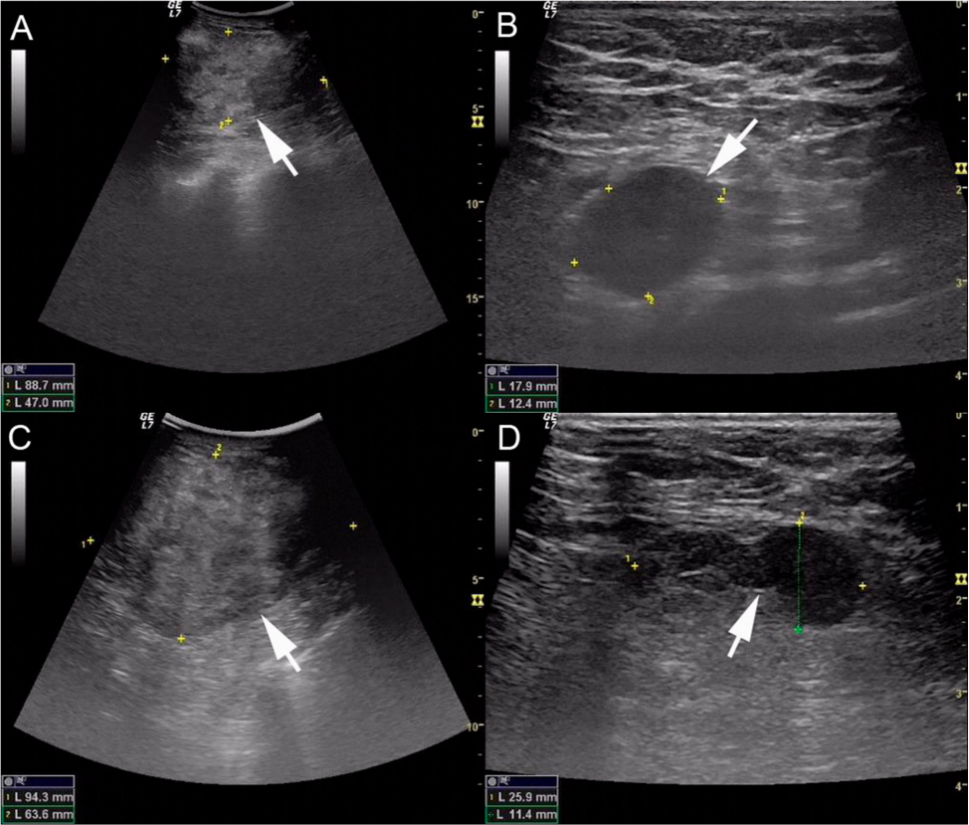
Ultrasonography of the breast. In 30 July 2014, ultrasonography revealed an 88.7-mm × 47.0-mm ill-defined heterogeneity lesion (arrow) in the upper inner quadrant of the right breast (A) with right enlarged axillary lymph nodes (arrow), 17.9 mm × 12.4 mm in diameter (B). In 21 August 2014, ultrasonography revealed a 94.3-mm × 63.6-mm ill-defined heterogeneity lesion (arrow) in the upper inner quadrant of the right breast (C) with right enlarged axillary lymph nodes (arrow), 25.9 mm × 11.4 mm in diameter (D).
